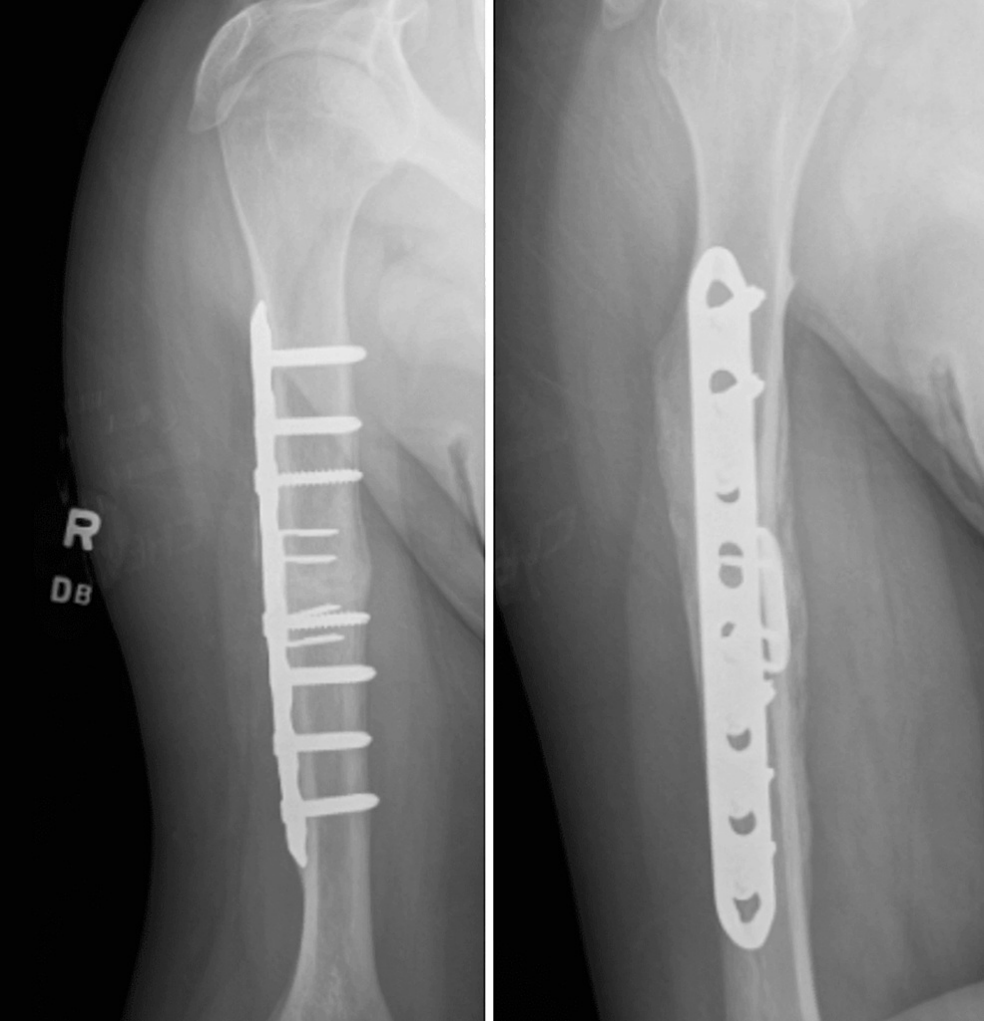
X-rays of the right humerus at eight months post-op. Complete healing of the fracture site is demonstrated.
